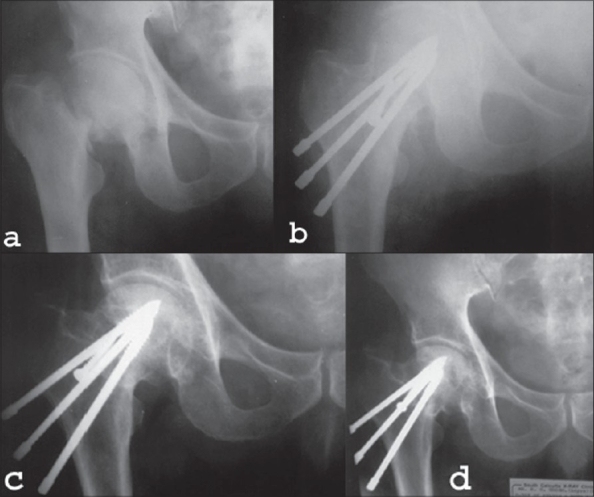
X-ray anteroposterior view (a) showing femoral neck fracture in an 81-year-old male patient (b) and immediate postoperative X-ray of the same patient. The muscle pedicle was fixed by a screw. (c) X-ray anteroposterior view of same patient showing six months follow-up (d) and five years follow-up. The pt showed excellent clinical outcome
